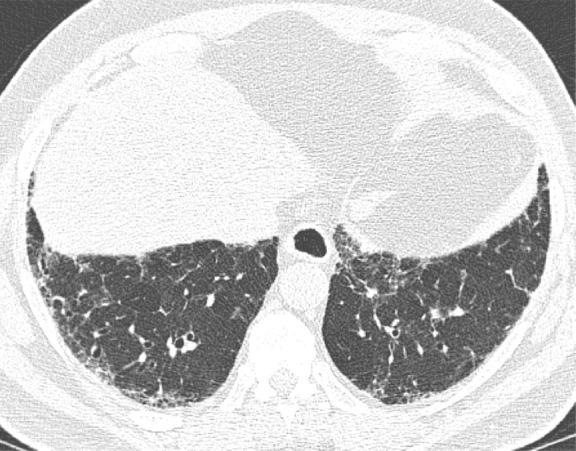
Probable usual interstitial pneumonia pattern. (a–c) Axial inspiratory CT images demonstrate peripheral and basilar-predominant reticular abnormality, with mild peripheral traction bronchiectasis or bronchiolectasis, but without associated honeycombing, making this a probable UIP pattern based on Fleischner and American Thoracic Society guidelines. (d) Coronal inspiratory CT scan confirms the lower lung–predominant distribution of disease in the craniocaudal plane.
